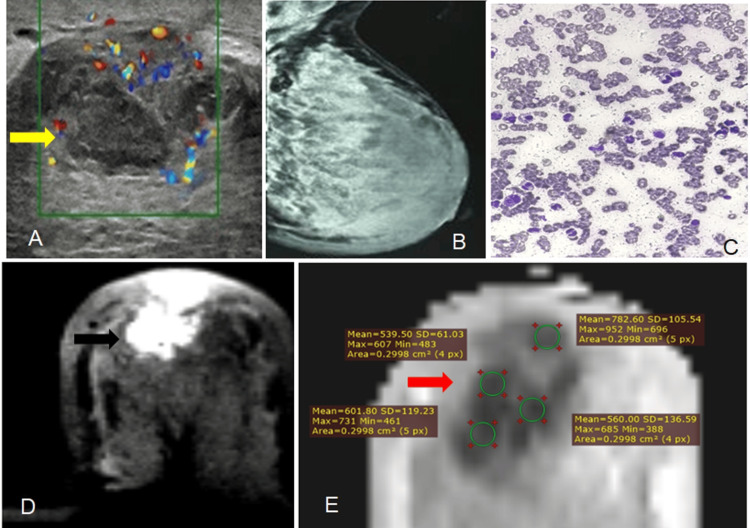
Mastitis (A) Ultrasound shows an irregular, vascular, heteroechoic space-occupying lesion (yellow arrow) with indistinct margins in a parallel orientation, surrounded by inflammatory changes, categorized as BI-RADS IVc.
